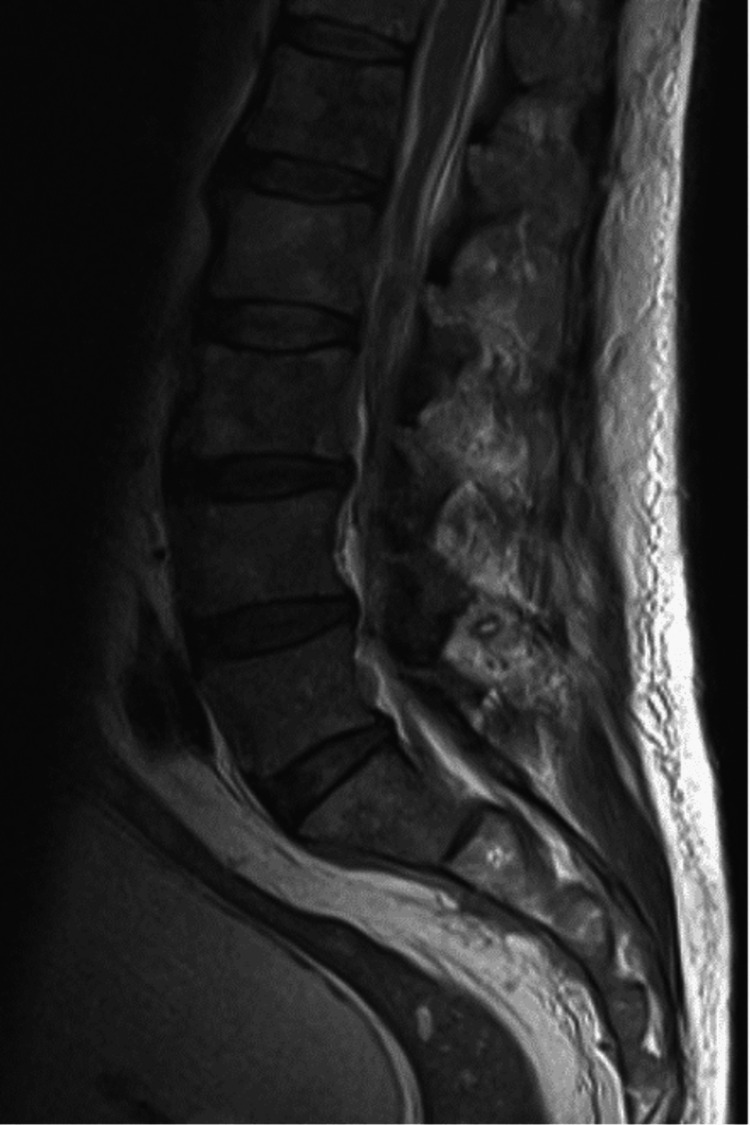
Magnetic resonance imaging performed in the emergency department suggesting bone marrow hyperplasia or tumor infiltration.
